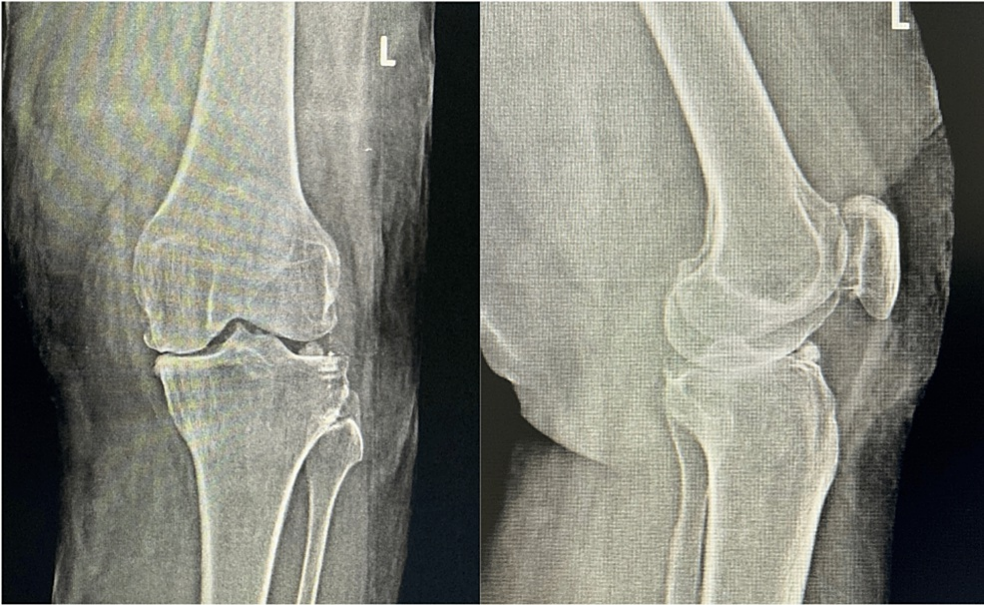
Standing AP and lateral radiographs of the knee revealed a loose body located anteriorly in the lateral compartment, alongside advanced osteoarthritic changes in the medial compartment.
